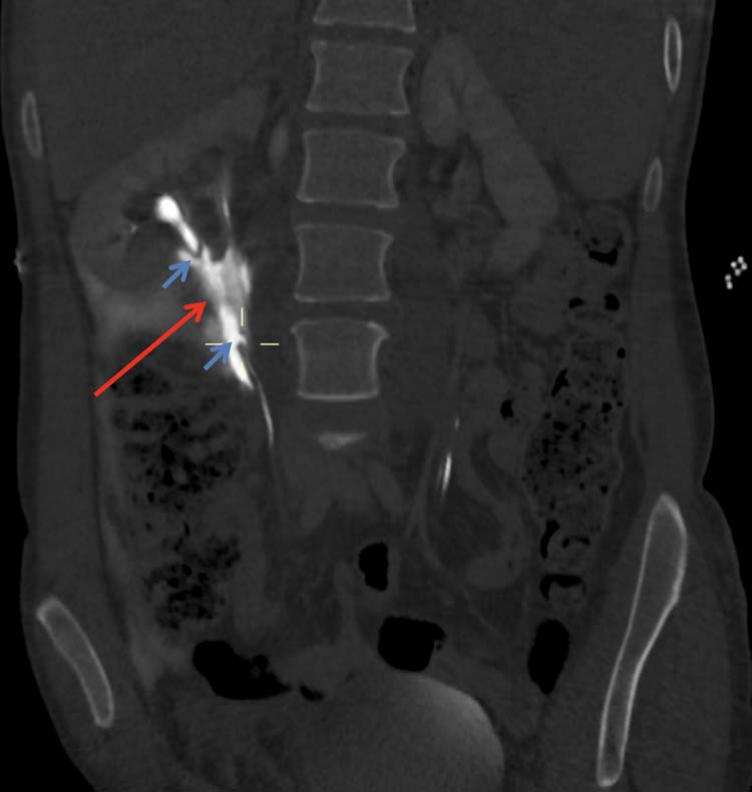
CT Urogram demonstrating extravasation of contrast. The blue arrows show the severed ends of the right inferior collecting system. The red arrow shows the contrast extravasation.
